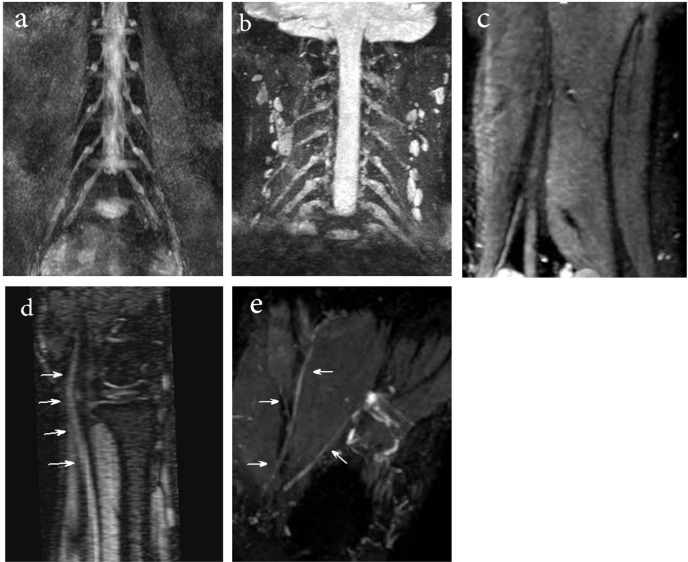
Thin section MIP reconstruction MR images, obtained with a 3D DW-SSFP sequence a: Lumbosacral plexus; b: Normal nerve root ganglia and proximal part of brachial plexus; c: Sciatic nerve and it branches; d: Median nerve; e: Medial and lateral plantar nerve
